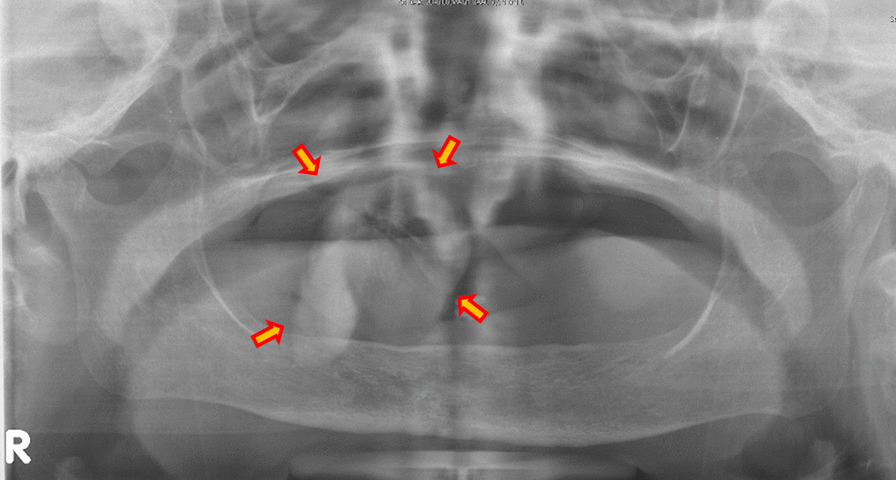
Panorama X-ray photograph revealing the shadow of the mass in the right maxillary premolar region, which includes some hard tissues (arrows)
